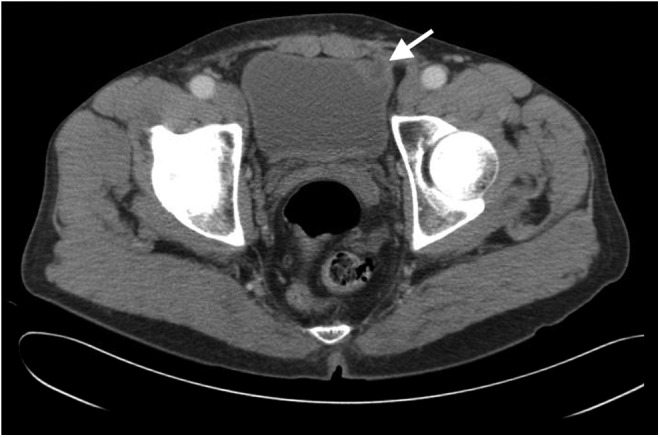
CT of the abdomen with evidence of abnormality at left anterior bladder wall indicated by *white arrow*.
